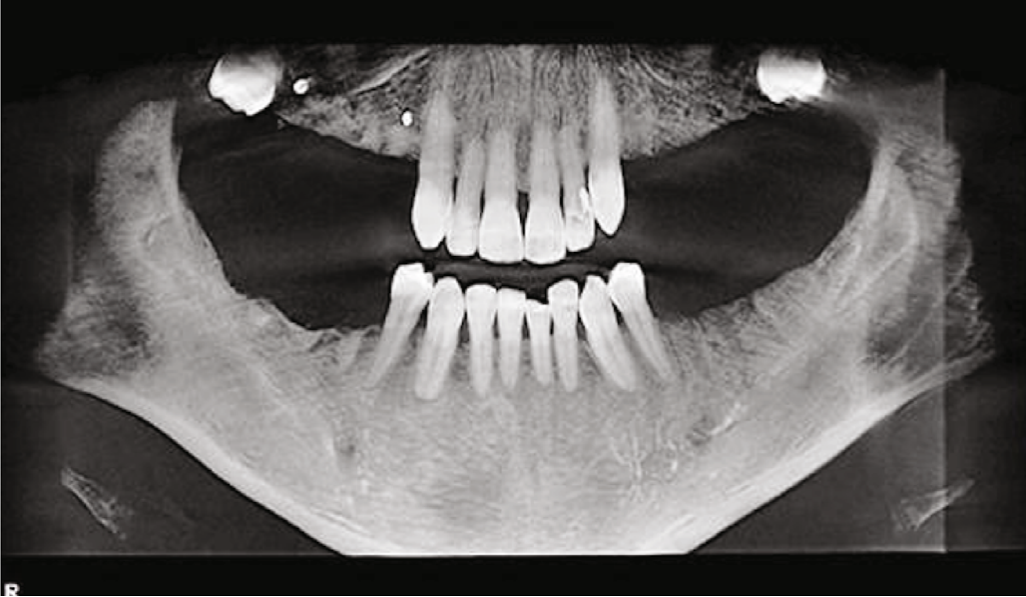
Panoramic radiograph (circa 2016) following extraction of all posterior teeth, except mandibular first bicuspids. Impacted third molars were not removed due to distinct possibility of ankylosis and potential for fracturing the posterior wall and floor of the maxillary antrum.
